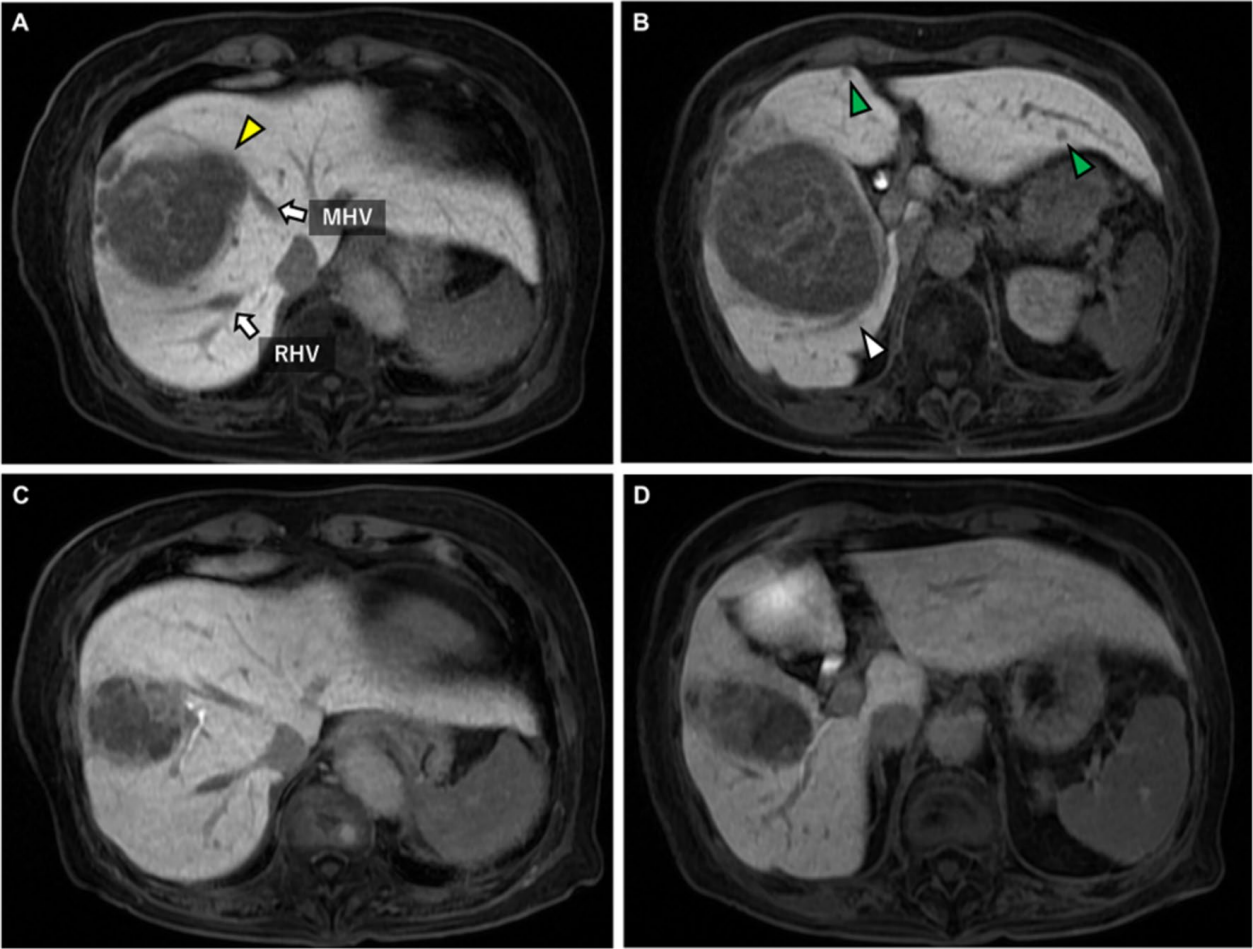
Magnetic resonance imaging (MRI). Huge hepatocellular carcinoma located in the hepatic anterior segment, before (A, B) and after (C, D) durvalumab plus tremelimumab treatment. A: The tumor caused compression and narrowing of the MHV (yellow arrowhead). B: Intrahepatic metastases in segments 3 and 4 of the liver (green arrowhead). Posterior segmental bile duct was dilated (white arrowhead). C: The tumor reduced in size. D: No intrahepatic metastases were found. *MHV* middle hepatic vein, *RHV* right hepatic vein
